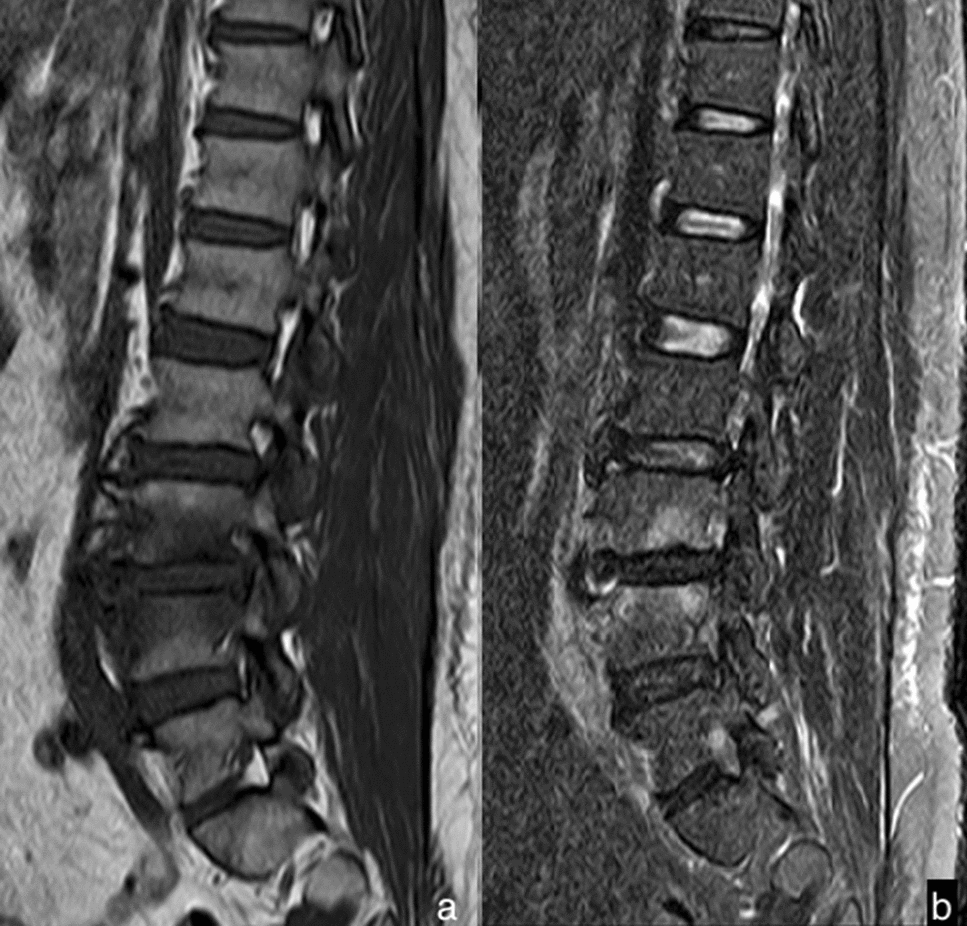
The MRI of a 47-year-old man suffering from weakness with brucella spondylitis. The lesions showed hypointense signal in T1WI (a) and slightly hyperintense signal in sagittal MRI with fat saturation (b) in lumbar vertebral bodies 4 and 5. Distinct vertebral hyperplasia and small abscesses could be found
